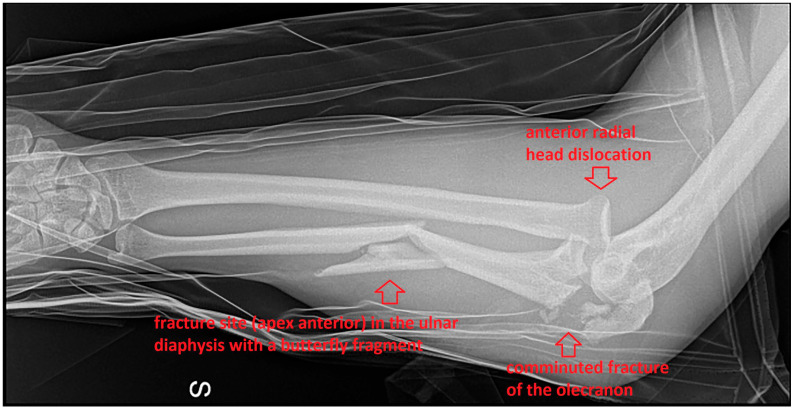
Initial radiograph of the Monteggia lesion.
